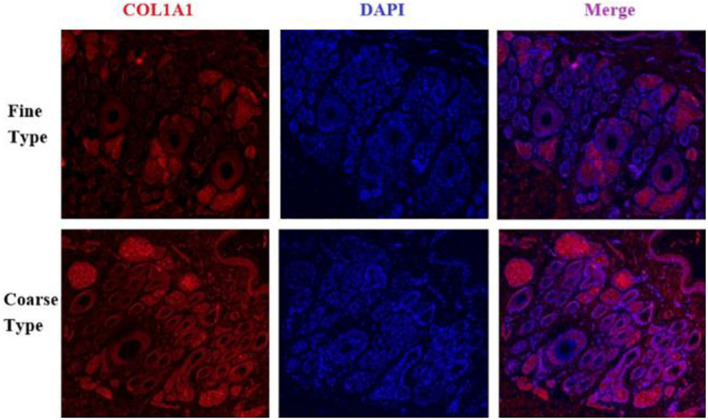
IF results of *COL1A1* in FT-LCG and CT-LCG skin tissues (40x).
